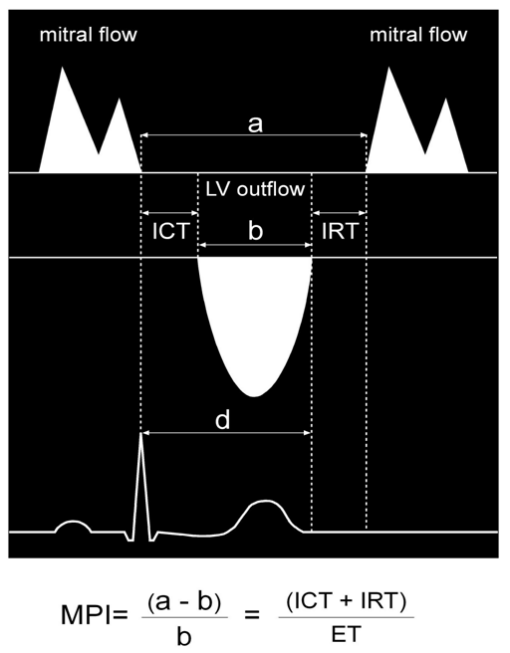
Flow Doppler at the mitral valve and at the LV outflow tract. Measurements of the time intervals of the Myocardial Performance Index (MPI). The interval "a" is measured from the end to the onset of mitral inflow waveform; the interval "b" is the left ventricular outflow velocity tracing (ET). The MPI was calculated as (a - b)/b.
